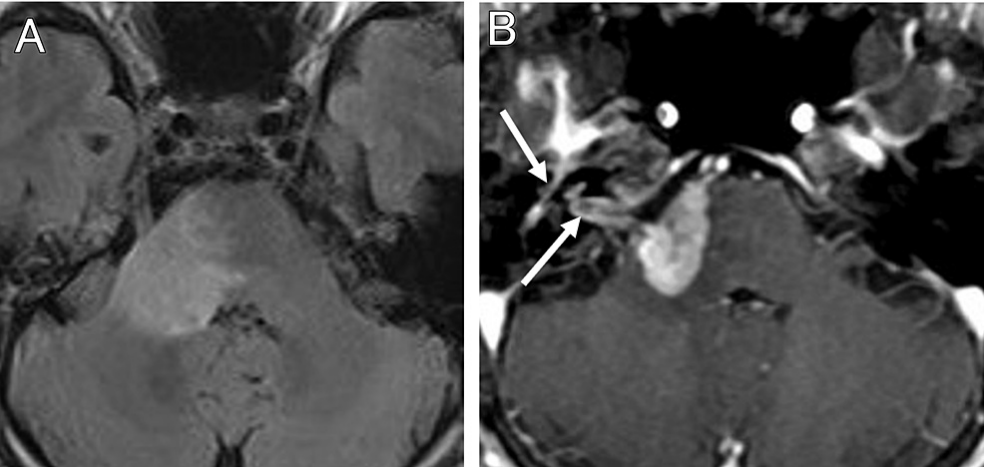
Follow-up MRI showing tumor invasion into the brainstem Two months after the treatment, FLAIR image (A), and contrast-enhanced T1-weighted image (B). The brainstem shows a mildly high-signal FLAIR image and a contrast-enhanced tumor on the right side. The tumor in the Gamma Knife-treated area has shrunk (arrows). MRI: magnetic resonance imaging, FLAIR: fluid-attenuated inversion recovery
